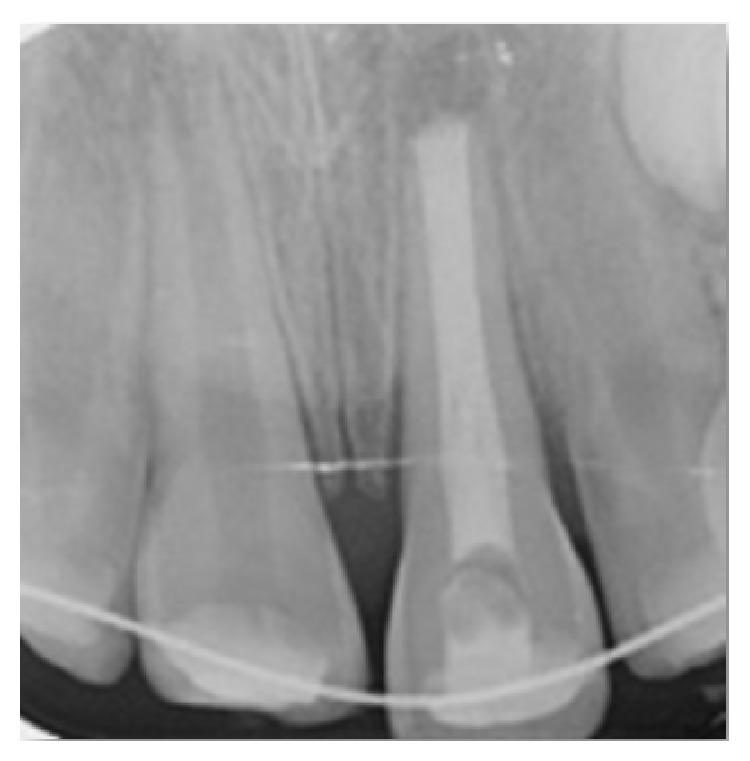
Periapical radiograph after immediate replantation of avulsed tooth.
